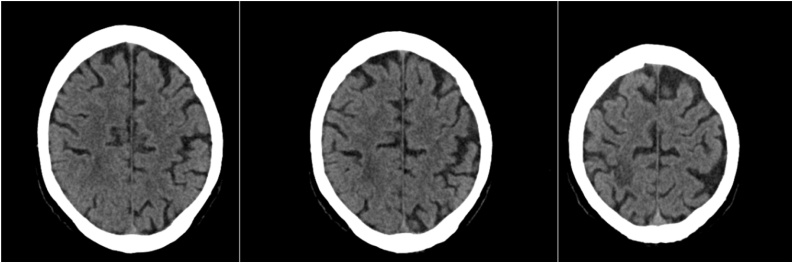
CT Scan with contrast a complete regression of the lesion and the surrounding edema.
